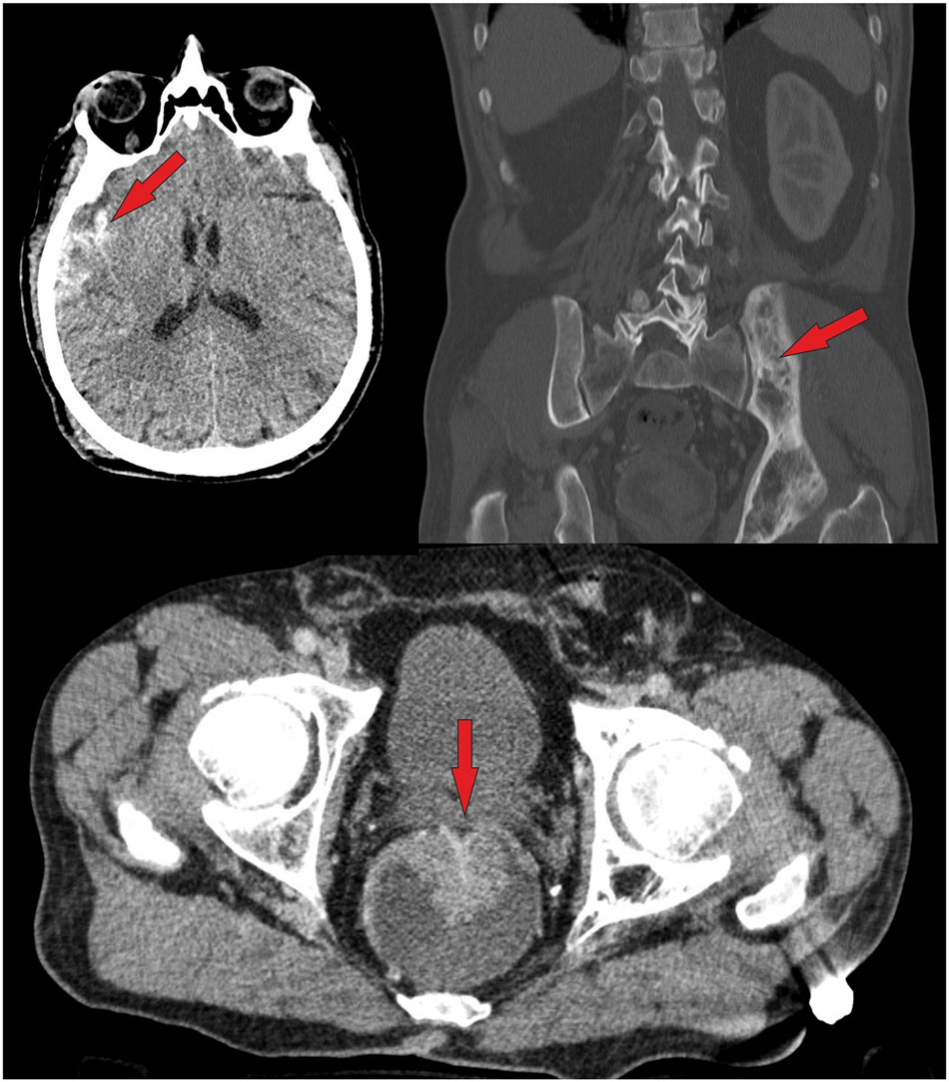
Patient with intracranial bleeding and some fractures of the extremities (shown in additional X-rays). IFs on whole-body CT scan: left pelvic fibrous dysplasia (IF 2, bone rads 1—benign lesion without aggressive features), and a suspicious mass in the rectum, classified (acc. to Table 1) as IF 5 because it was interpreted as a carcinoma
